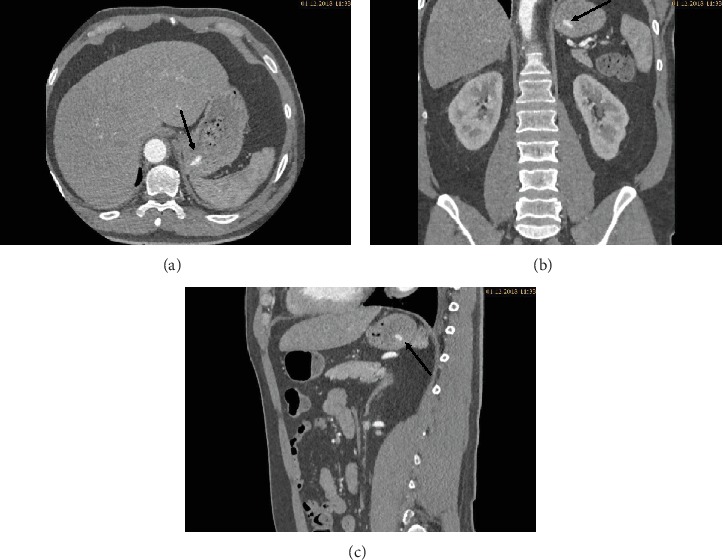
(a–c) Transversal, coronal, and sagittal slides of the abdominal CT in the arterial phase: a faint hyperdense lesion is seen in the posterior fundus of the stomach.
